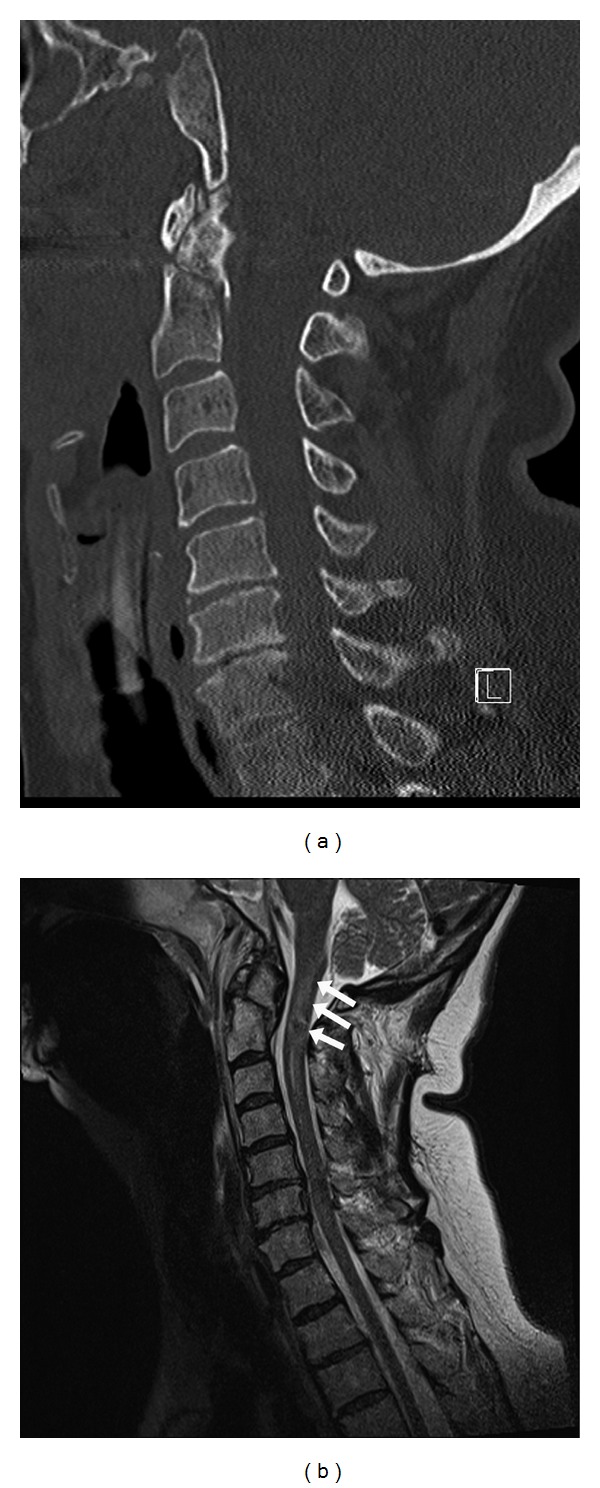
(a). Sagittal CT scan demonstrating type III odontoid fracture. (b). Sagittal nonenhanced T2-weighted MRI showing a cervical cord contusion with abnormal signal from the lower medulla to the inferior level of C3 (arrows).
